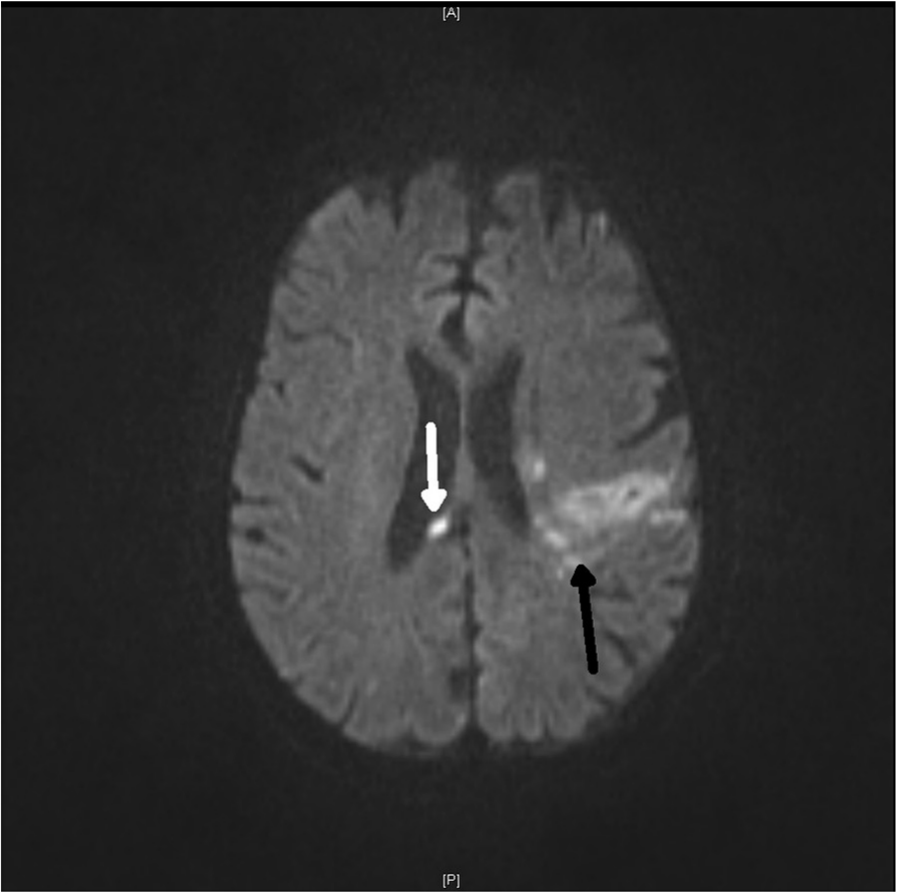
MRI head shows a left temporal infarct (black arrow) and a small R sided periventricular infarct (white arrow). Findings consistent with embolic stroke
